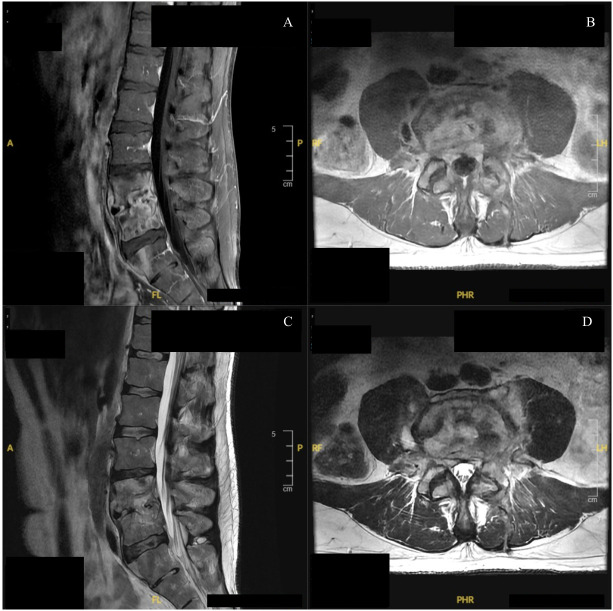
Initial presentation MRI showing chronic L4–L5 spondylodiscitis and abscess. MRI on presentation demonstrating chronic L4–L5 spondylodiscitis with enhancing endplate necrosis and drainage into the disc space. Infection extends to the L5–S1 disc with anterior S1 osteomyelitis and suspected involvement of the L3–L4 disc space. Bilateral psoas and left paraspinal abscesses are present, with a 4 mm L4–L5 abscess/phlegmon and extension of inflammation into the bilateral L4–L5 and L5–S1 neural foramina. (A) Sagittal T1 post-contrast MRI, (B) axial T1 post-contrast MRI, (C) sagittal T2 image, and (D) axial T2 image. Alt text: MRI of the lumbar spine demonstrating chronic L4–L5 spondylodiscitis with endplate destruction, disc space involvement, and extension to L5–S1 and possibly L3–L4, along with bilateral psoas and left paraspinal abscesses and inflammatory involvement of adjacent neural foramina (sagittal and axial T1 post-contrast and T2 images).
